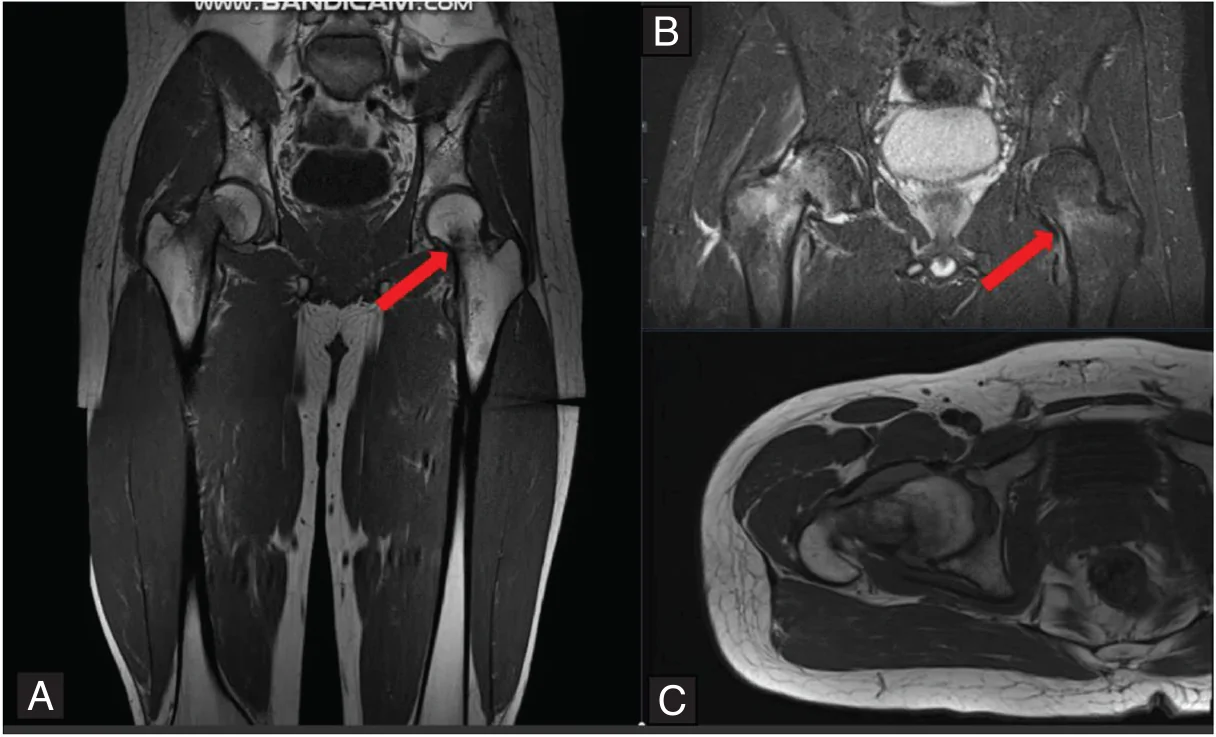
(A) MRI hypointense signal changes over the left neck of femur in T1W image. (B) MRI hyperintense signal changes over the left neck of femur in T2W STIR image. (C) MRI axial cut of right hip showing hypointense signal changes over the neck of femur.
